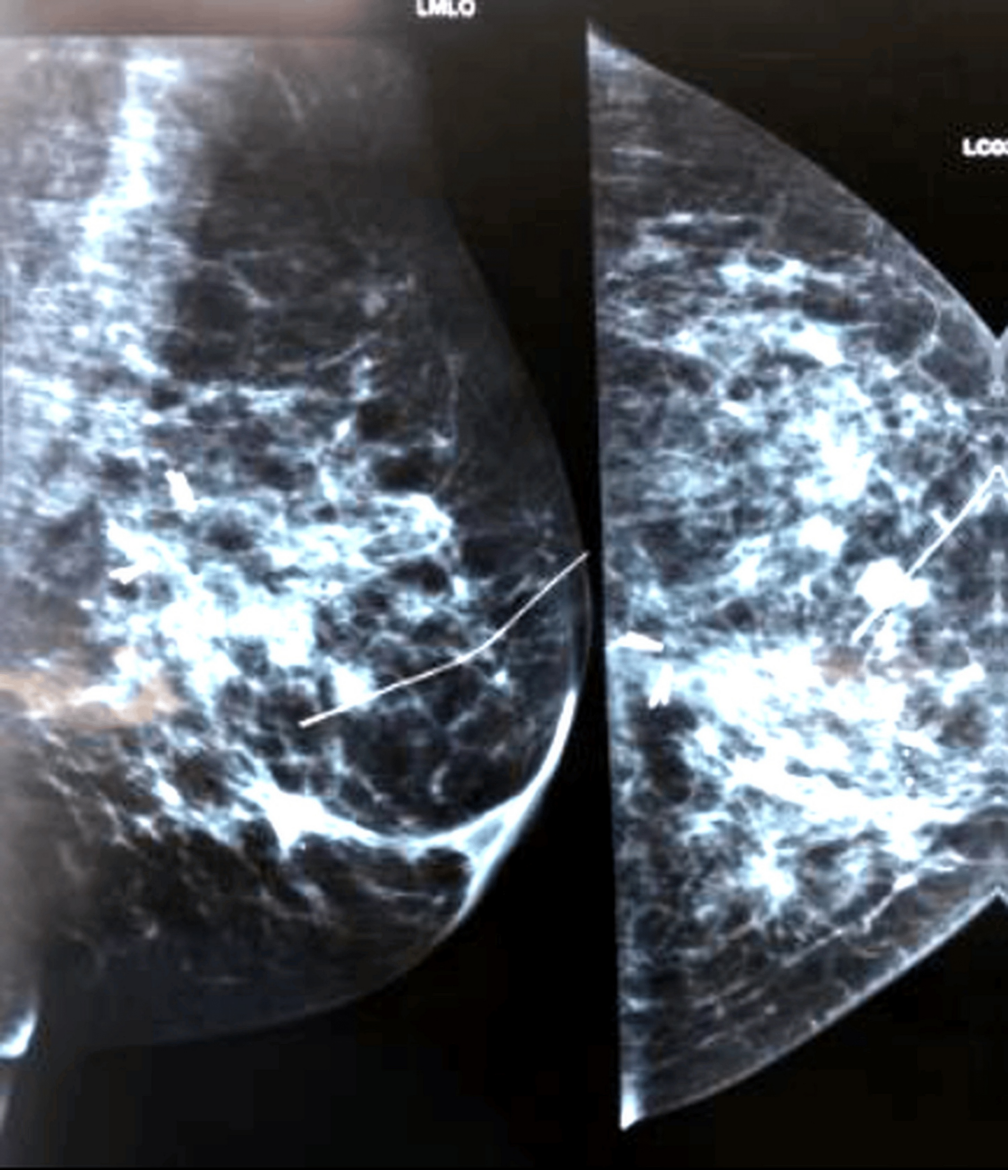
Post-ultrasound-guided wire insertion mammogram The wire is transfixing the lesion.
